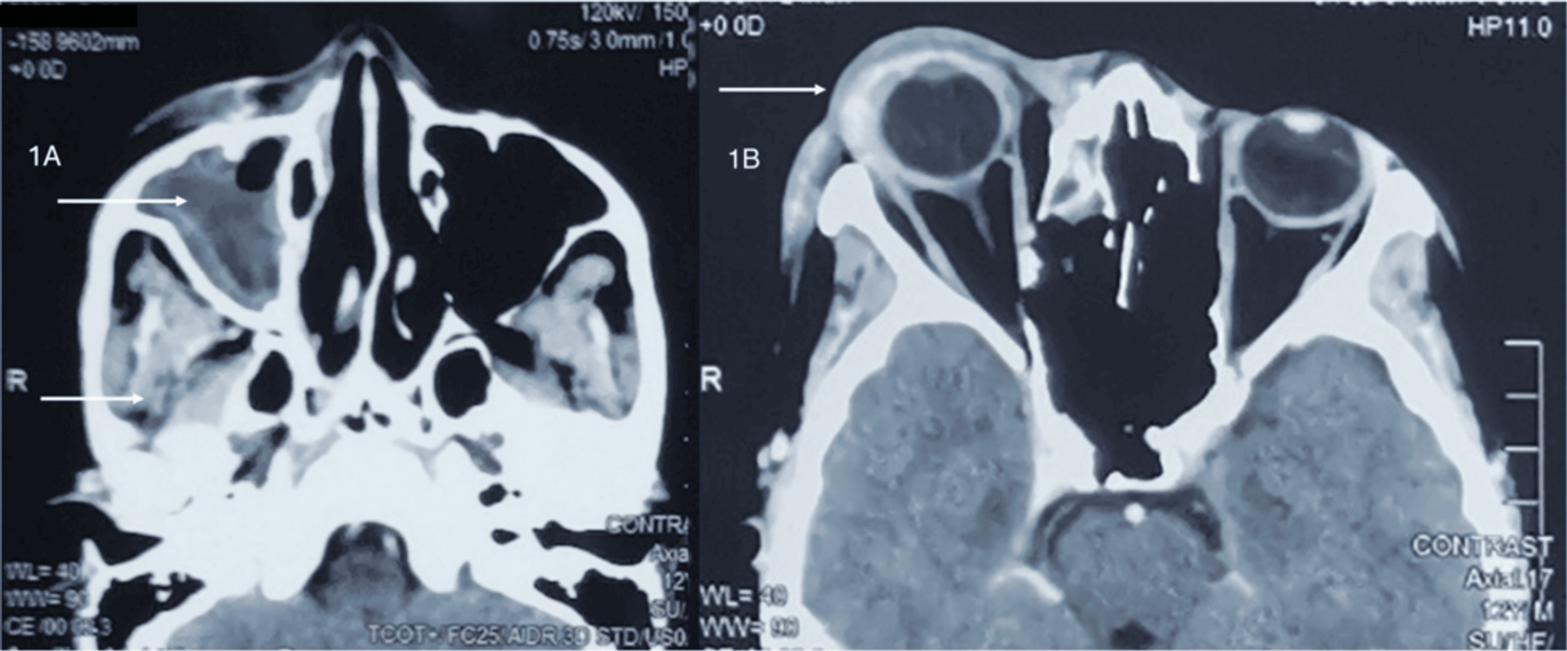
Axial section of the orbital CT scan revealing a large, multiseptated abscess in the right superior extraconal region, opacification of the right maxillary sinus (1A), and proptosis of the right globe (1B).
